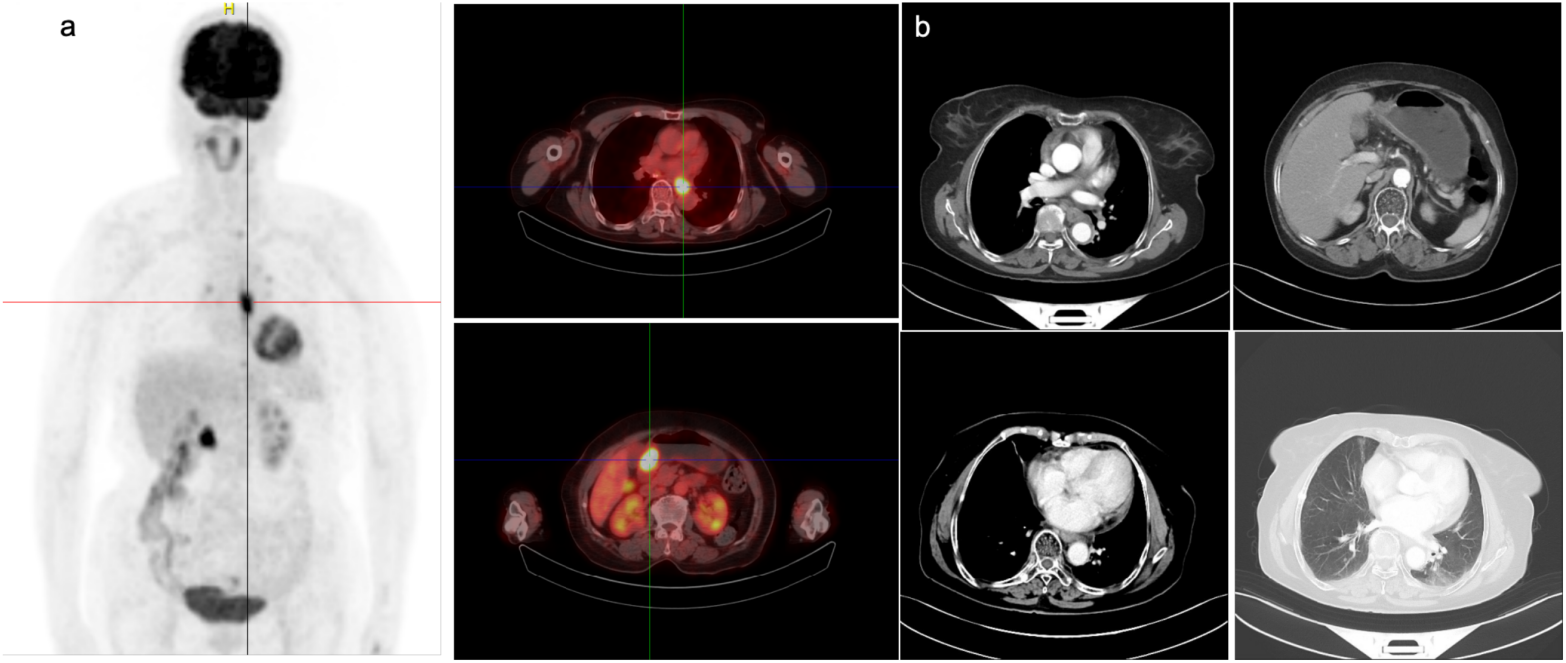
Imaging findings of the patient before and after admission. (a) PET-CT imaging on March 31, 2022, showed localized thickening of the mid-thoracic esophageal wall with a maximum SUV of 13.4. Slightly increased uptake was observed at the proximal and distal ends of the esophagus, as well as near the gastroesophageal junction, with a maximum SUV of 4.0. Thickening of the gastric antrum wall was also noted, with a maximum SUV of 15.1. (b) CT scan on September 21, 2022, showing no significant change in the esophageal cancer after treatment compared to the previous scan.
